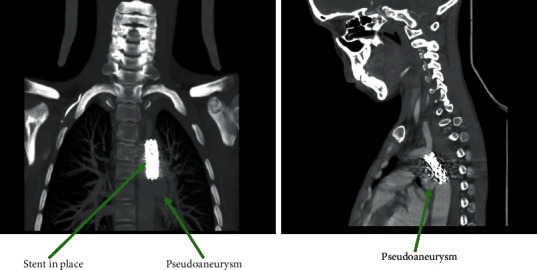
The thoracic angio-CT scan revealing a good opacification of the ascending aorta and the aortic arch with stent in place, with two sacciform pseudoaneurysms in the descending aorta just below the stent.
